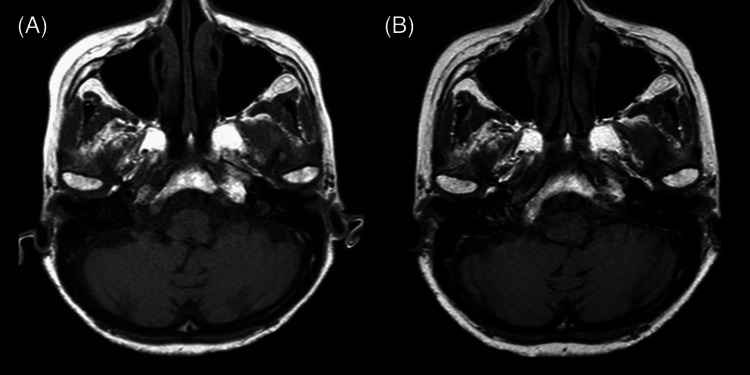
Magnetic resonance images T1-weighted magnetic resonance images at the upper levels of the cerebellum on admission (A) and two years after tuberculosis treatment (B). Neither image shows swelling, atrophy of the cerebellum, or other abnormal signals.
